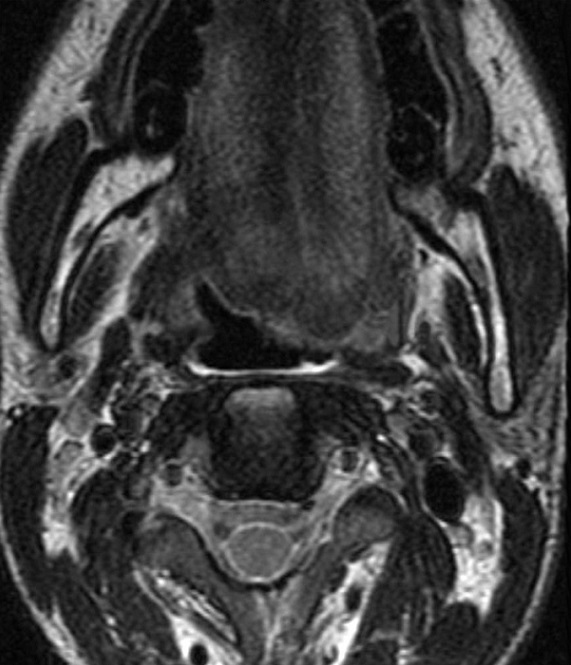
MRI on T2 weighted image showing the drooping and flabby aspects of the left oropharyngeal wall and the left deviation of the tongue
